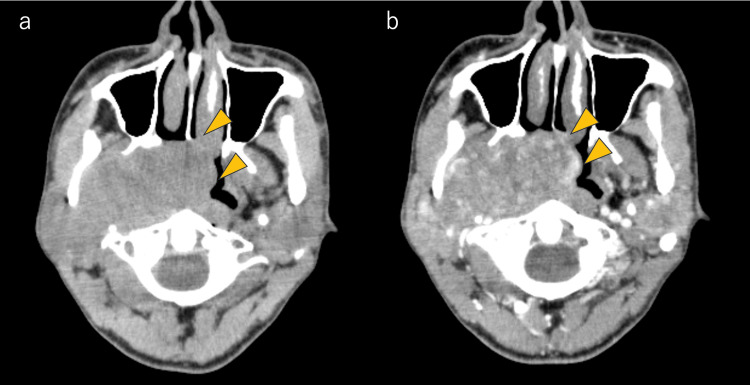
Images of computed tomography performed at the first visit Compared with a plain CT (a), the contrast-enhanced CT (b) revealed a lesion of 65 mm in diameter, indicating internal contrast heterogeneously (arrowhead).
